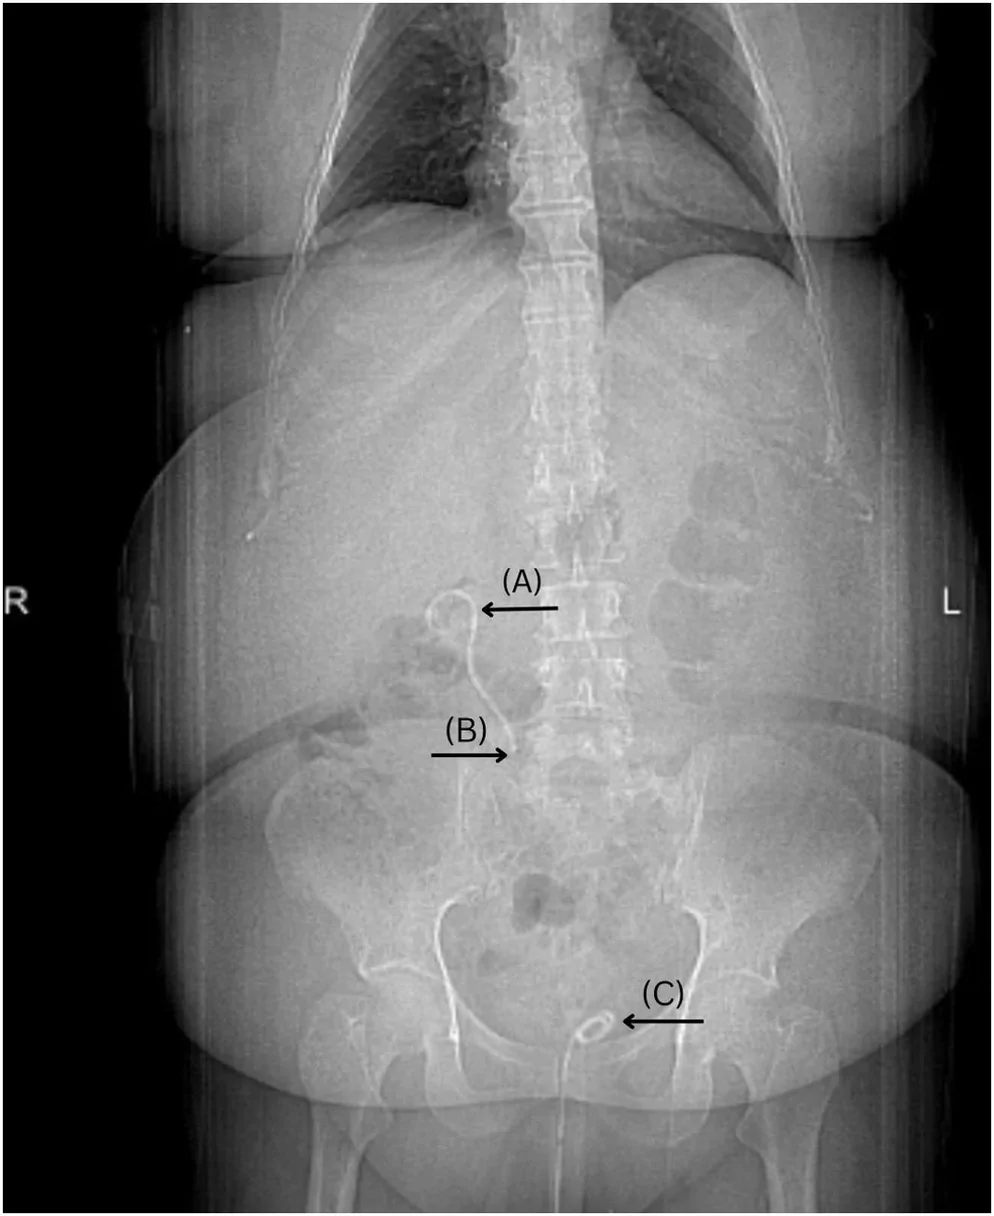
Kidney, ureter, and bladder (KUB) radiograph demonstrating a fragmented right‐sided DJ stent. (A) Proximal coil located within the right renal pelvis. (B) Discontinuity along the ureter consistent with stent fragmentation. (C) Distal coil visualized within the urinary bladder.
